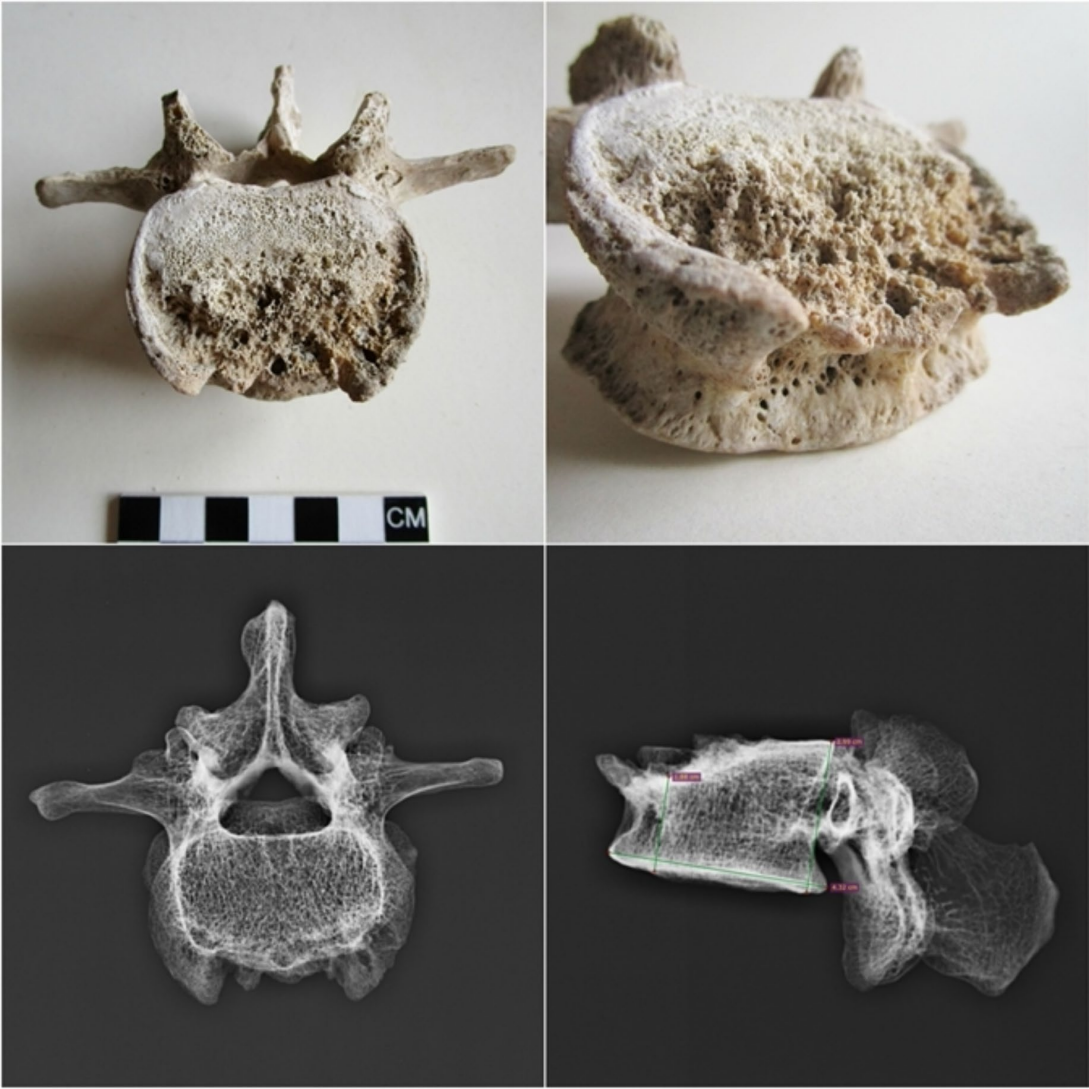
Photography and digital radiograph of the fourth lumbar vertebra (L4) affected by tuberculous spondylodiscitis of the individual A.
